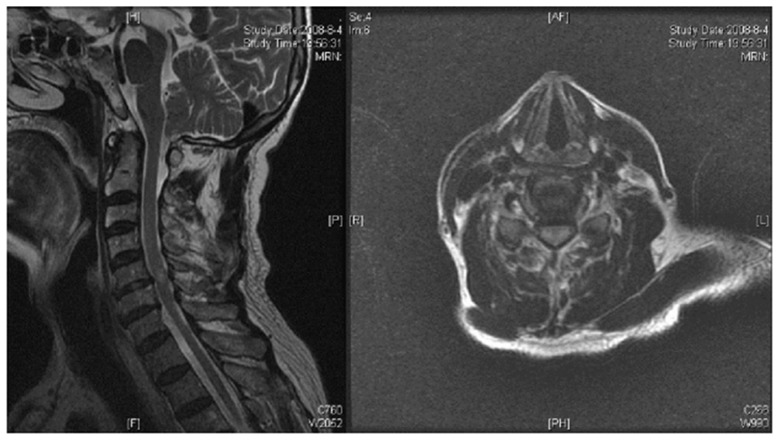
Case 1. Sixty-eight-year-old male exhibiting disc herniation at the C5–6 level. T2-weighted magnetic resonance imaging (MRI) revealed high signal changes in the spinal cord.
